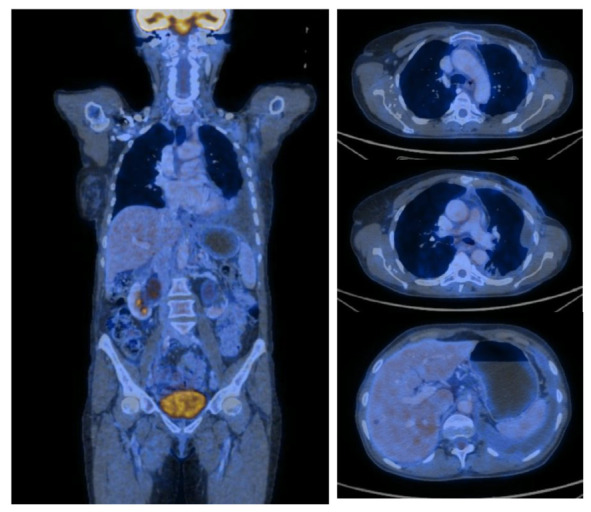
PET-CT from June 2021 with no metabolically active lesions.
